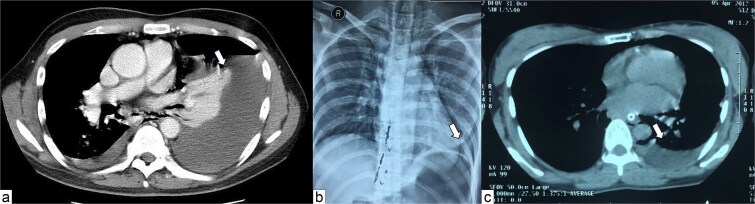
Chest X-ray and CT scan of the patients. (a) Marked pleural effusion (arrow) on contrast-enhanced CT scan of the first patient. (b) Chest X-ray findings of the second patient, note that there was unclear pleural effusion on initial X-ray (arrow). (c) Non-contrast CT findings of the second patient, with marked pleural effusion (arrow).
